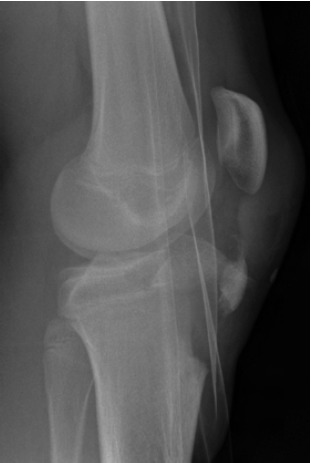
Preoperative lateral radiograph of the left knee.
